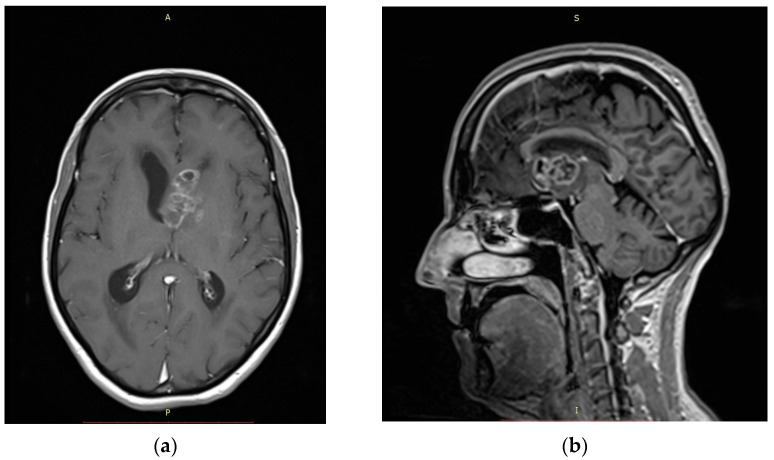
(a) Axial plane T1-weighted gadolinium-enhanced MRI scan of patient with glioblastoma located in the head of the caudate nucleus included in our study. (b) Sagittal plane T1-weighted gadolinium-enhanced MRI scan of the same patient.
